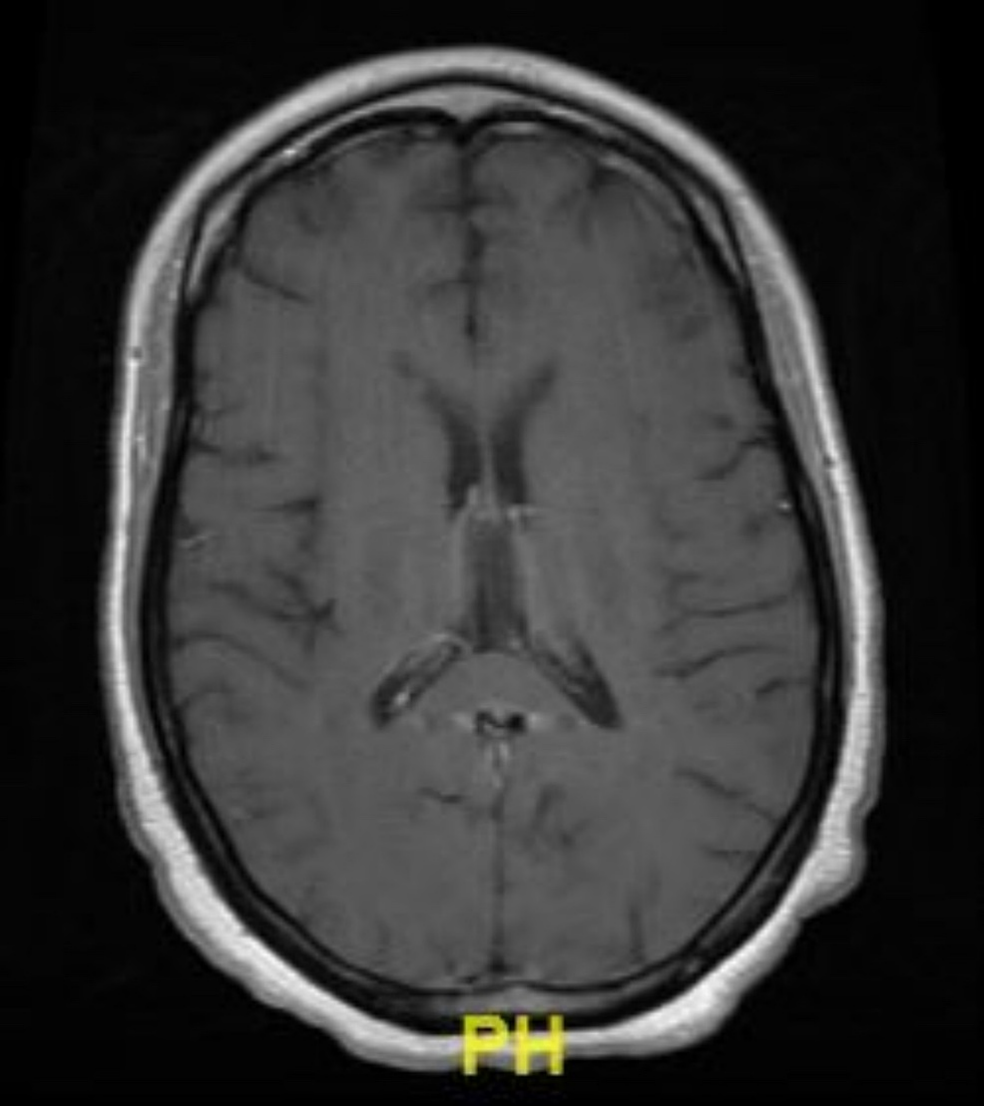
Magnetic resonance imaging with and without contrast with no acute infarction, hemorrhage, or mass.
